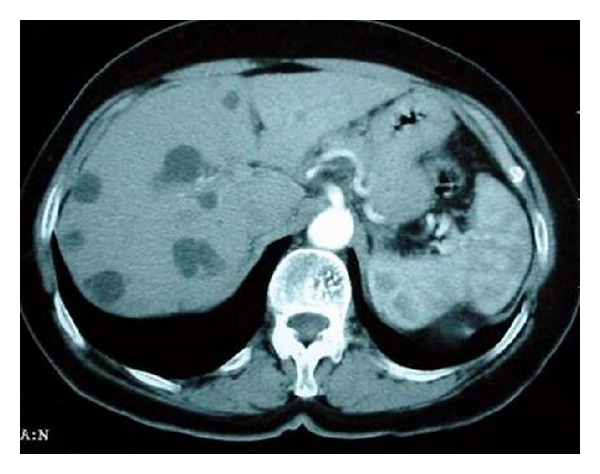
Abdominal and pelvic CT scan in a patient with hepatorenal polycystic disease. Large hepatic cysts causing stenosis and displacement of the inferior vena cava and compression of the suprahepatic veins in relation to a Budd-Chiari syndrome.
